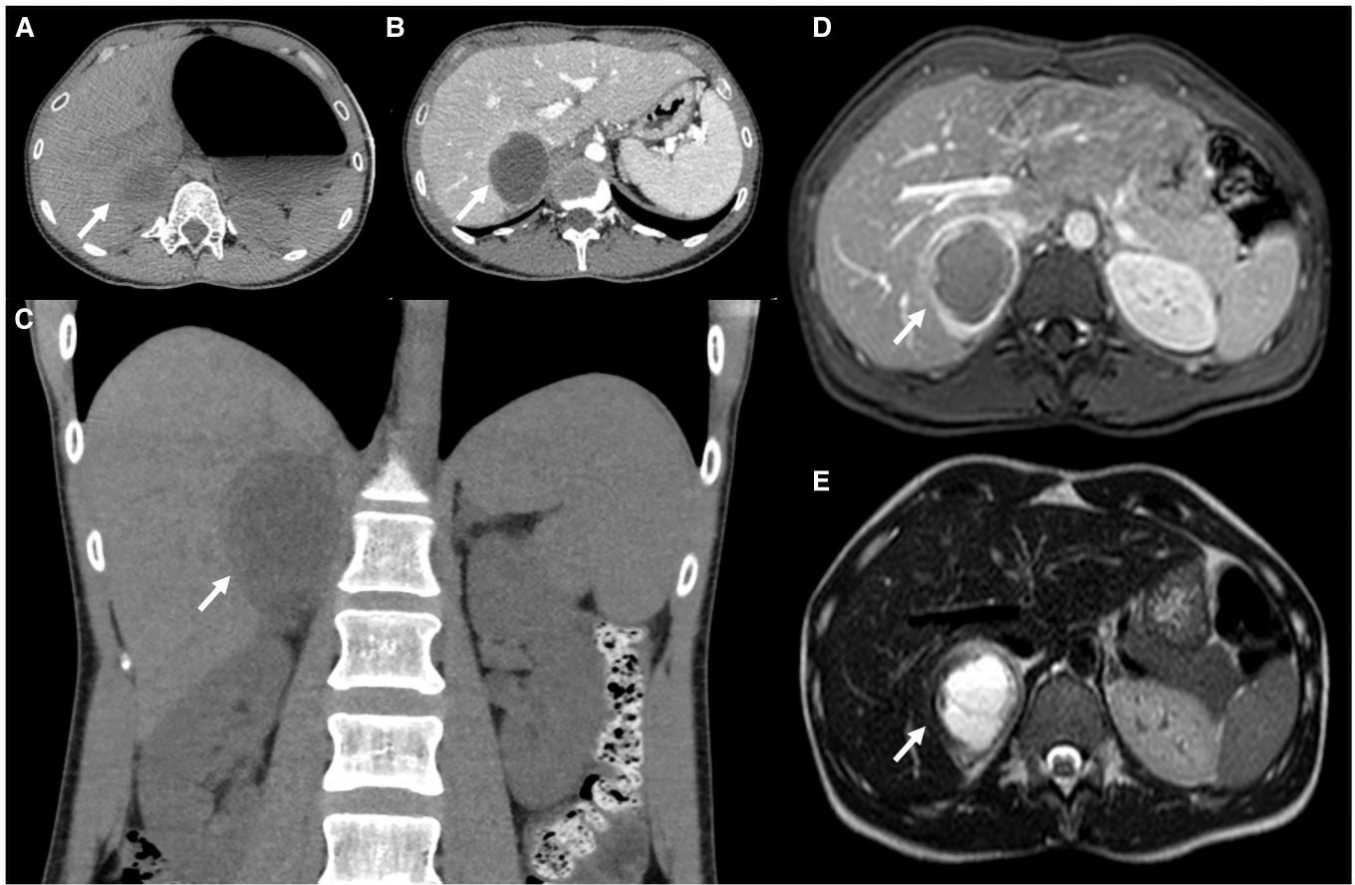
Abdominal computed tomography and magnetic resonance imaging. Axial computed tomography showing a large right adrenal mass measuring 7.1 × 6.6 cm (arrow) in sequences without contrast (*A*) and with contrast (*B*) with evidence of recent bleeding; coronal reconstruction (*C*) in computed tomography addressing the tumour (arrow); magnetic resonance imaging in T1 (*D*) and T2 (*E*) sequences showing the pheochromocytoma (arrow) with almost complete haemorrhagic necrosis.
